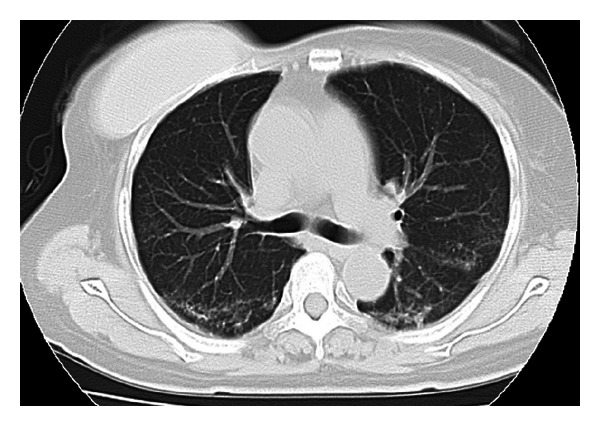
Computed tomography after the treatment for *pneumocystis pneumonia*. CT showing that pulmonary infiltration has almost disappeared as of 8 days after initiation of therapy.
